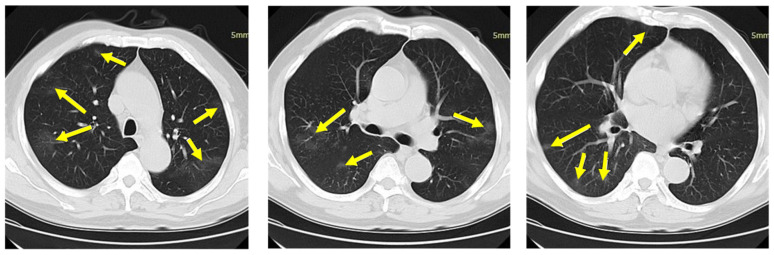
All CT images of a single CT case are shown here. The center of infection in the image is indicated by arrows.
